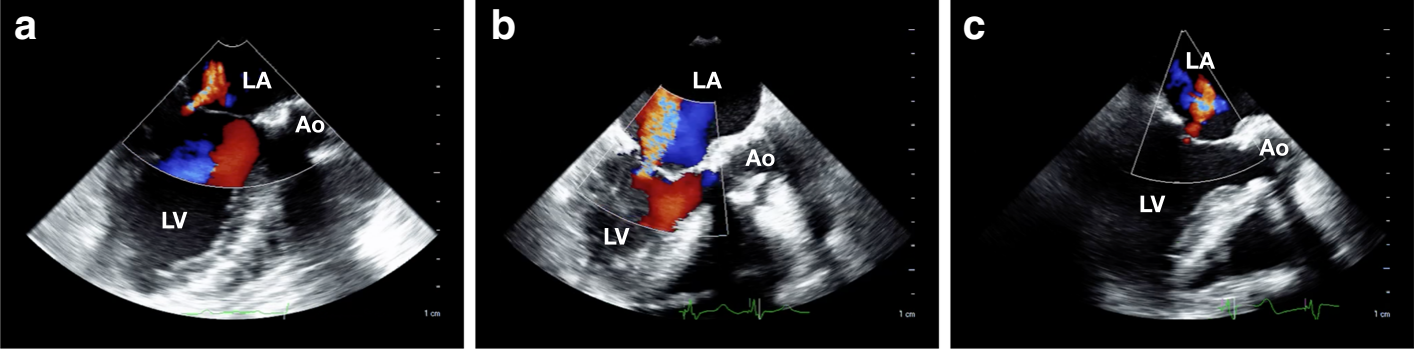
Midesophageal, long-axis, two-dimensional transesophageal echocardiography view of the mitral valve. A mild centrally directed mitral regurgitation (MR) due to compromised coaptation with a vena contracta of 2.3 mm was demonstrated before initiating cardiopulmonary bypass (CPB) (a). Following the first surgical aortic valve replacement (AVR), severe centrally directed MR with restriction and distortion of the anterior mitral leaflet was revealed (vena contracta, 8.6 mm), while there was no evident injury of the anterior mitral leaflet, ventricular enlargement, or myocardial hypokinesis (b). After re-AVR, there was no restriction or tethering of the anterior mitral leaflet and the MR was reduced to the same mild degree (vena contracta, 2.5 mm) as that before initiating CPB (c). LA, left atrium; LV, left ventricle; Ao, aorta
